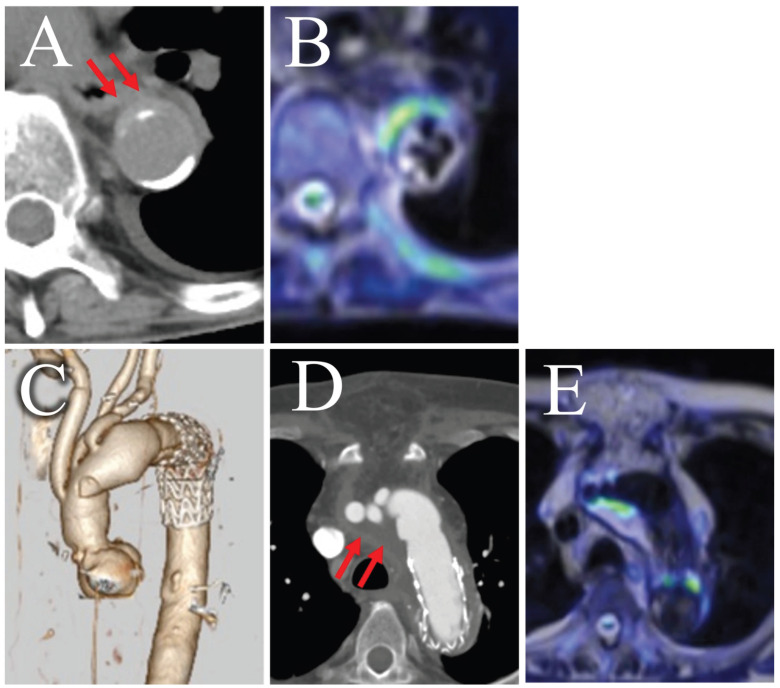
Pitfalls of DWIBS. Accurate diagnosis requires integrating multiple imaging modalities. Relying solely on MRI, especially DWIBS and T2-weighted imaging, may not suffice for comprehensive assessment. (A,B) A case of a thrombosed aortic dissection. (A) Axial NCCT (soft-tissue window); (B) axial DWIBS (b = 800 s/mm^2^) fused with T2-weighted imaging. In (A), the false lumen (arrows) shows high attenuation on NCCT, whereas in (B), it appears hyperintense on DWIBS. Differentiating these features using DWIBS alone is challenging, but CT values facilitate an accurate distinction. (C–E) Early postoperative images following surgical repair of an infectious aortic aneurysm. (C) A 3D reconstruction from CE-CT; (D) an axial CE-CT image that shows no enhancement around the graft but reveals a soft-tissue shadow (arrow); (E) DWIBS fused with T2-weighted imaging, demonstrating partially high signal intensity around the graft. However, based on clinical findings and follow-up, no evidence of infection was found. Detailed imaging parameters: DWIBS (b = 800 s/mm^2^, slice thickness: 6 mm); CT (slice thickness: 1 mm, soft-tissue kernel). In the fused images (B,E), the blending ratio of DWIBS to T2-weighted images was adjusted to ensure accurate localization. DWIBS signal intensity is displayed in pseudo-color, where red indicates high signal intensity and blue indicates lower signal intensity. DWIBS, diffusion-weighted whole-body imaging with background suppression; MRI, magnetic resonance imaging; NCCT, non-contrast computed tomography; CE-CT, contrast-enhanced computed tomography; CT, computed tomography.
